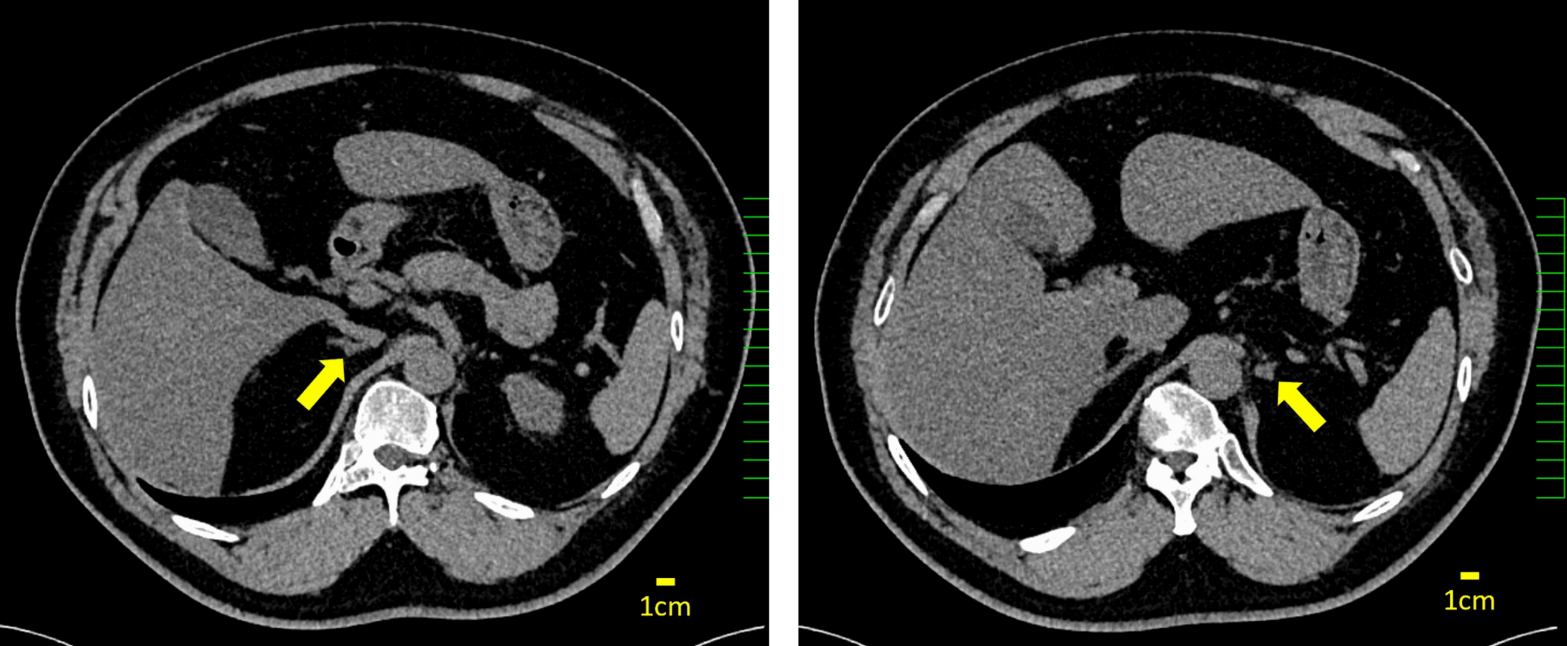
Axial computed tomography scan demonstrating normal adrenal glands. The figure shows the periadrenal area with the adrenal glands marked by yellow arrows. Both adrenal glands measured less than 10 mm in body thickness (normal range for adrenal body: right 7.2±1.8 mm, left 8.8±1.9 mm), with all measurements falling within normal reference ranges [5]. This finding supports the exclusion of adrenal hyperplasia as a contributing factor to hypokalemia.
